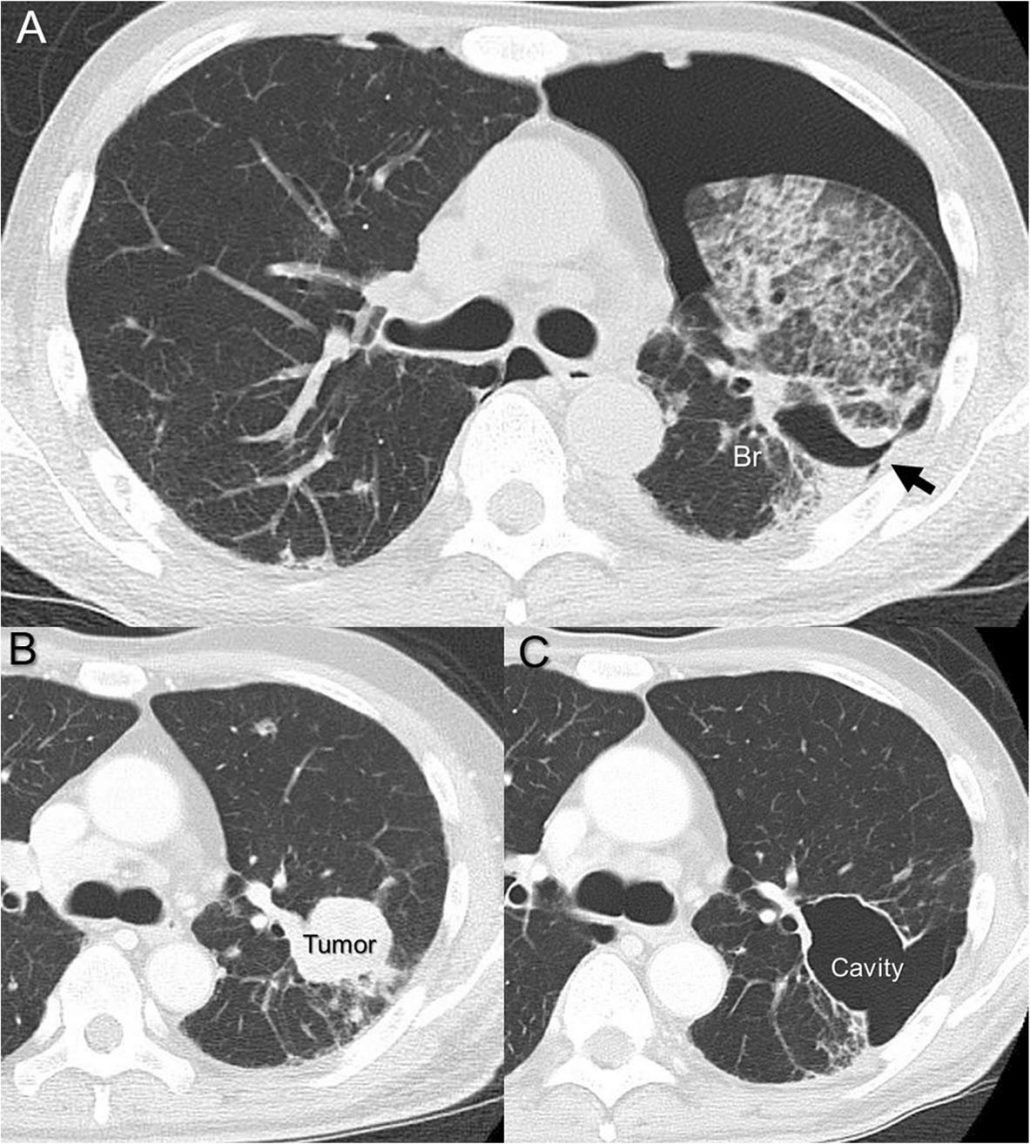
Chest computed tomography (CT) revealing left pneumothorax and a pleural defect (black arrow) in the left upper lobe (LUL) (a). CT before axitinib therapy initiation showing a nodule in the LUL (b). CT after the therapy showing a cavity that developed at the same site as the tumor (c). Br, bronchus
